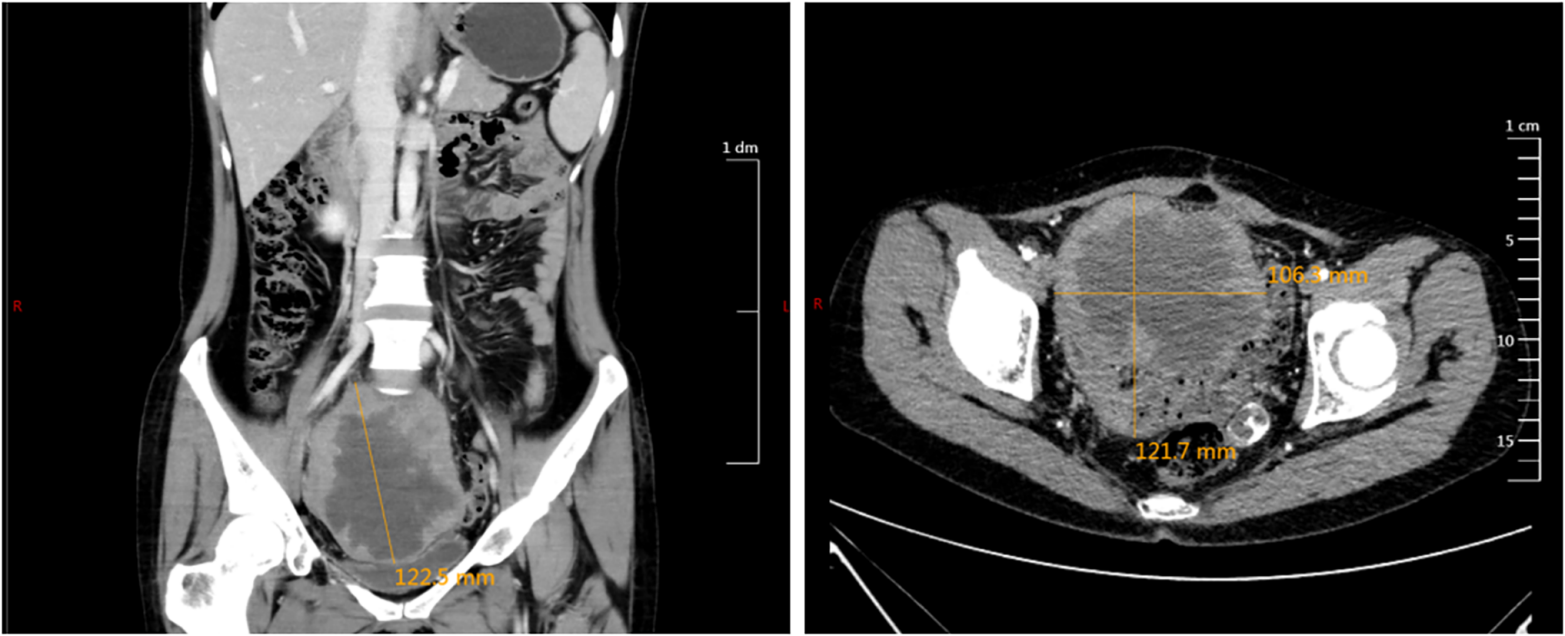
CT scan prior to the second operation. Hepatogastric space, mesenteric space, the largest of multiple soft tissue nodules in front of the right psoas major muscle at the level of the fifth lumbar vertebra, on the left side of the pelvic floor was approximately 13.6*11*9.4 cm in size, which was uneven and partially accompanied by calcified density.
